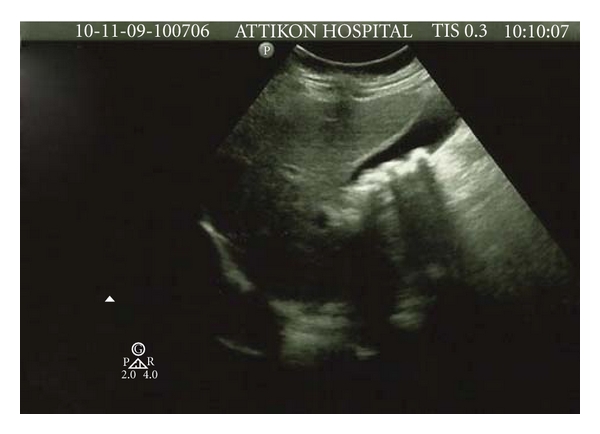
Sonogram in one of our patients showing multiple echogenic material with acoustic shadowing.
